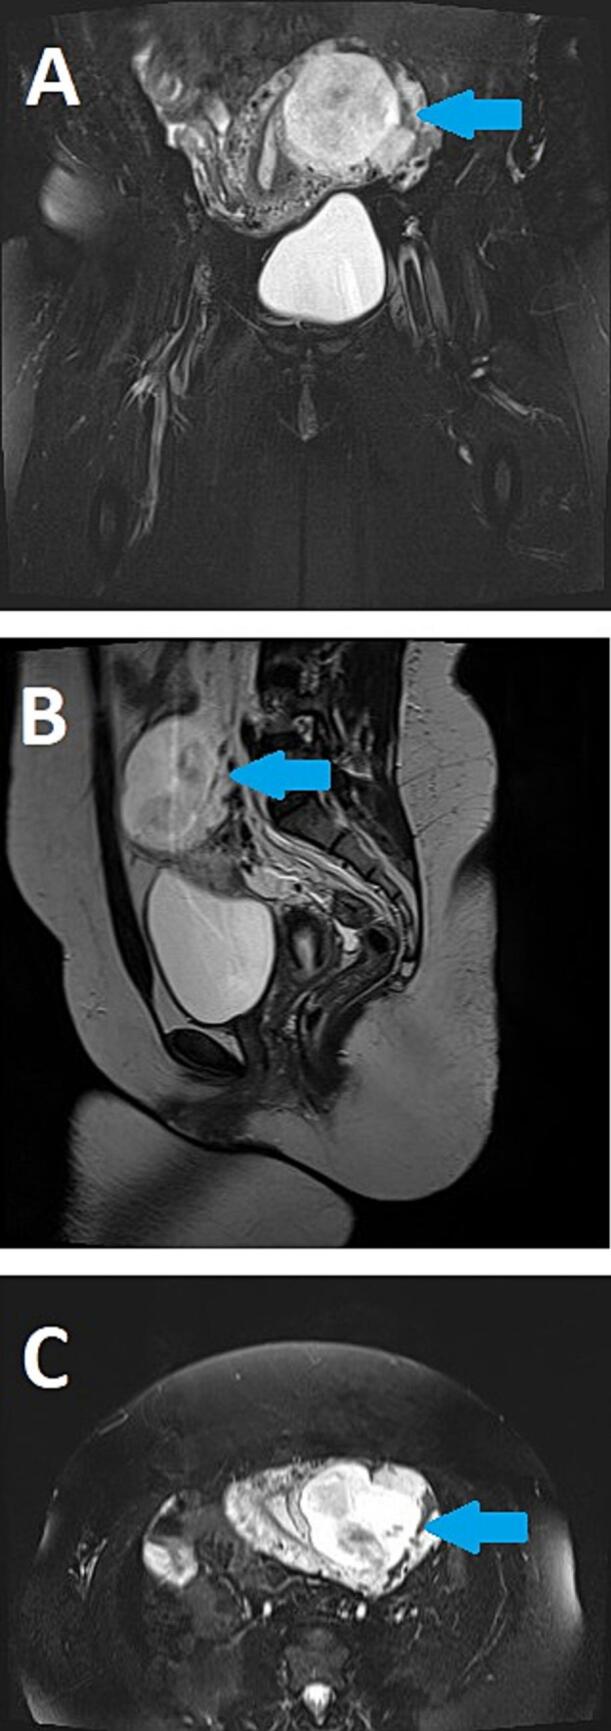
An MRI pelvis. A – Coronal view showing a heterogeneous signal intensity mass (Blue arrow) centered at the interstitial portion of the left fallopian tube immediately lateral to the left uterine angle. B – Sagittal view of a mass with a fetus within (blue arrow). C – Axial view of a mass on the left side with a fetus within (blue arrow).
